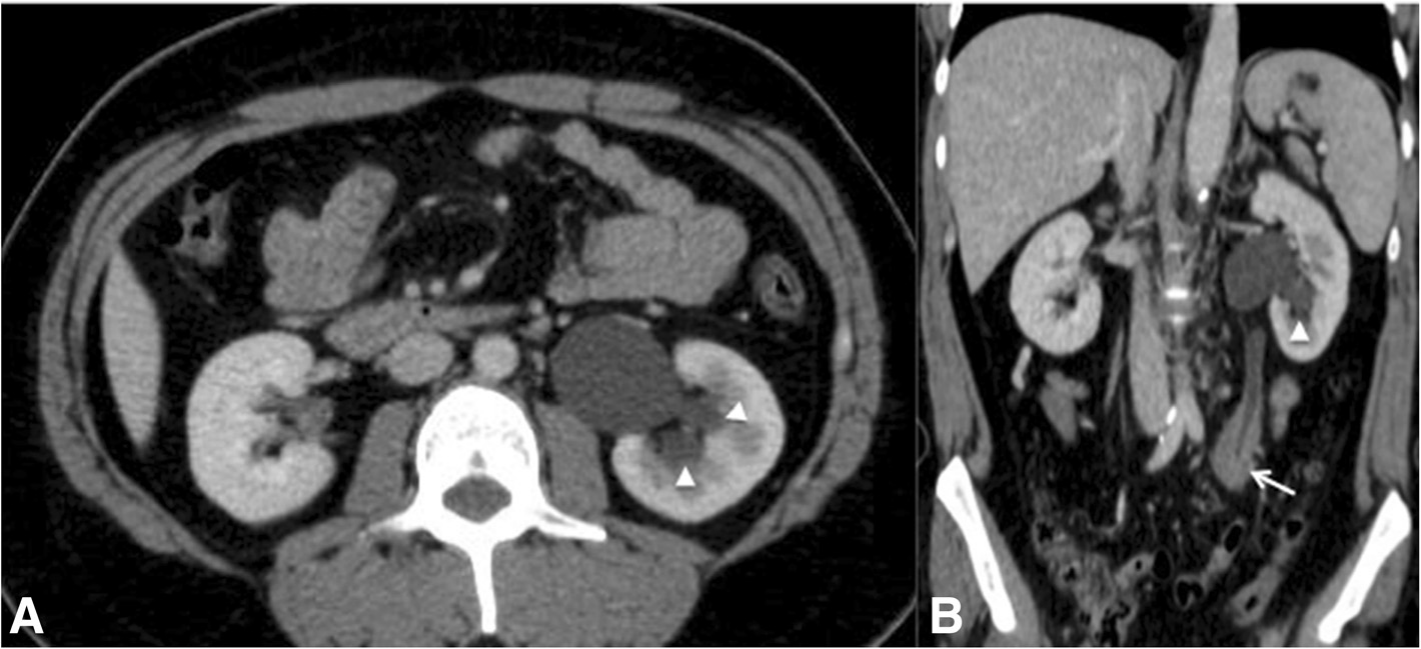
A 23-year-old man with urinary obstruction. (A) Axial and (B) coronal CT images of the abdomen obtained after intravenous contrast administration showing mild hydronephrosis (arrowhead) on the left, with a discrete delay in the concentration of intravenous contrast by the left kidney caused by a soft-tissue density mass (lymphoma) involving the middle third of the left ureter (arrow).
